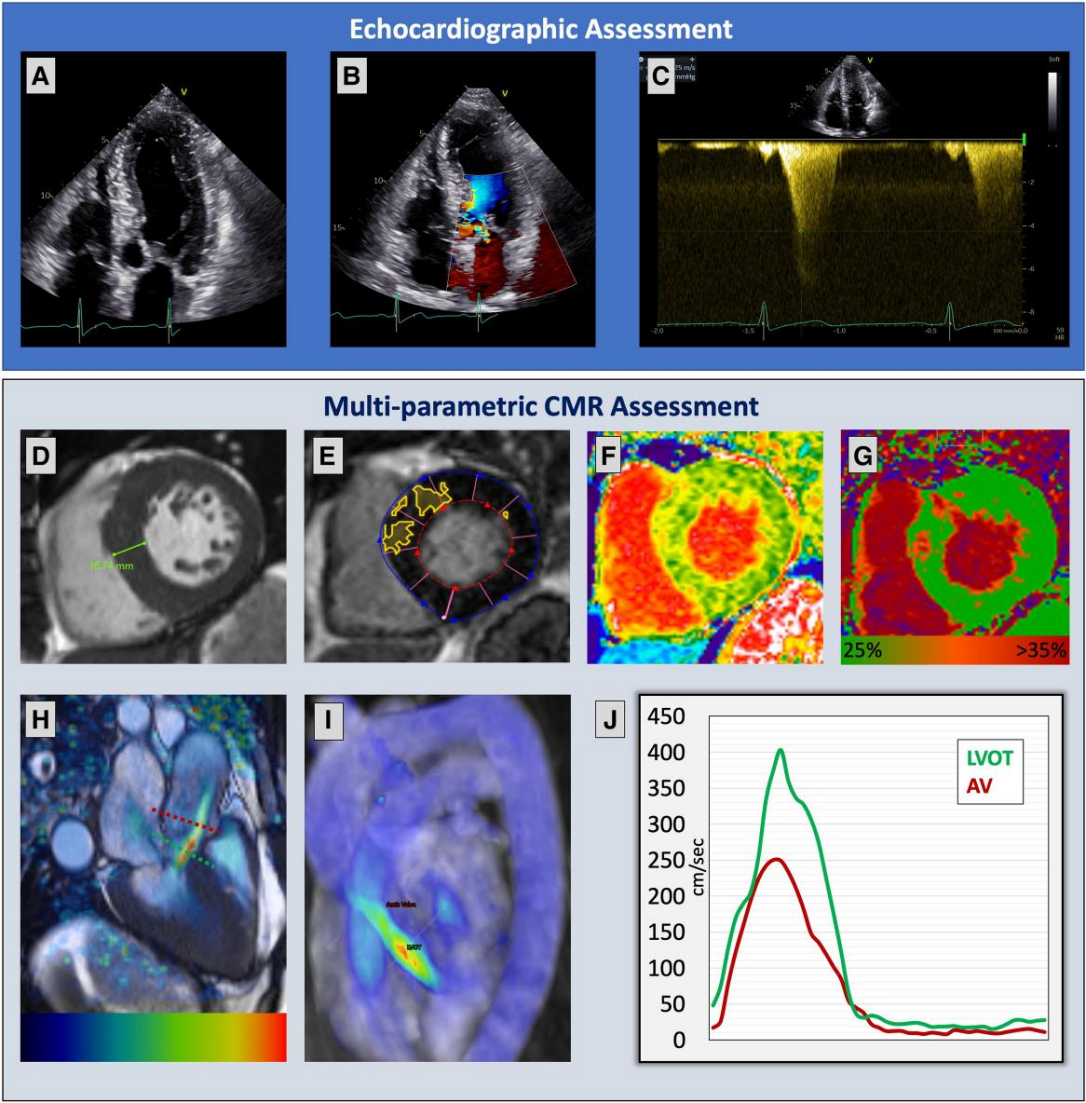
Echocardiographic and multi-parametric cardiovascular magnetic resonance imaging assessment of aortic stenosis. (*A*–*C*) Apical four-chamber long-axis view demonstrating the systolic anterior motion of the anterior mitral valve leaflet towards the outflow tract and possible flow acceleration on colour-Doppler. The peak velocity on continuous wave Doppler was 4.25 m/s. However, due to the mitral regurgitation jet superimposing the outflow tract flow, it was not clear if this was indeed in the outflow tract. Importantly, as this was not a pulse wave Doppler, it was difficult to determine the spatial location of the peak velocity. (*D*) Steady-state free precession short-axis cine images showing asymmetrical septal hypertrophy (16.74 mm at end-diastole). (*E*) Late gadolinium enhancement imaging with a predefined signal threshold of 3 SD, demonstrating mid-wall myocardial fibrosis. (*F* and *G*) Native and post-contrast T1 mapping demonstrating fibrotic changes in the anterior wall and septum, consistent with the diagnosis of hypertrophic cardiomyopathy. (*H*–*J*) Four-dimensional flow three-dimensional velocity visualization of flow and its speed demonstrate that the flow acceleration is happening in the left ventricular outflow tract with a peak velocity of 4.07 m/s. The systolic anterior motion can be seen in (*H*) clearly with resultant mitral regurgitation.
